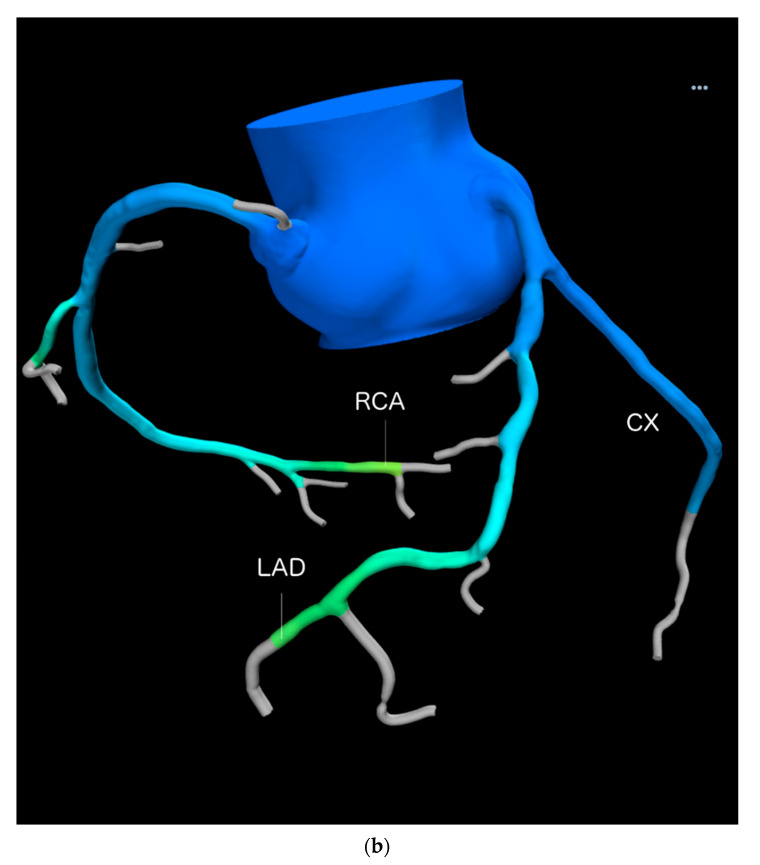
(a) 22-year-old professional male endurance athlete (swimmer) with regular high training volumes and a new onset of high-grade arrhythmia. Enlarged proximal but smaller mid and distal RCA (upper) and LAD (lower panel) segments (“*athlete’s artery*”). Quantitative CTA (right) included sizing of RCA and LAD dimensions at 3 sites (proximal, mid and distal) using curved multiplanar reformations (cMPR). Both vessel area (mm^2^) and 2 diameters (mm) were taken. Left panels show volume rendering technique (VRT). CX = circumflex artery. (b) 3D Quantification of vessel volumes (mm^3^) for the RCA, LAD and CX by using a computational fluid modelling (CFD) software (Heartflow, Inc. Redwood, CA, USA) in another patient.
